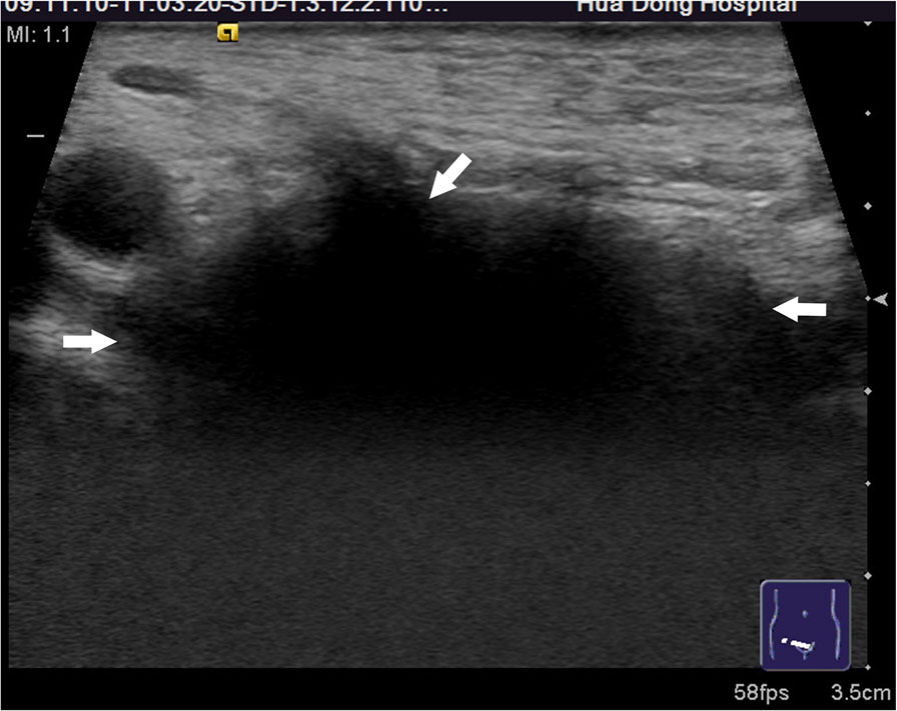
Accumulational meshes showed in the ultrasonography
